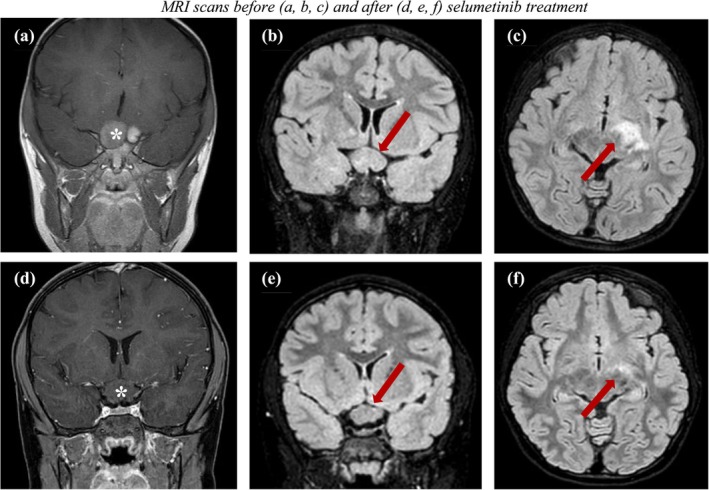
Pre‐selumetinib MRI: (a) coronal post‐contrast T1‐weighted, (b) post‐chemotherapy coronal and axial and (c) axial FLAIR images. Figure (a) demonstrates post‐contrast enhancement of the optic tract lesion; figure (c) shows signal hyperintensity of the optic tract. Two years after starting selumetinib: coronal contrast‐enhanced T1‐weighted (d), coronal FLAIR (e) and axial FLAIR (f) images demonstrate almost complete resolution of the left optic tract FLAIR hyperintensity, along with a slight reduction in the size of the optic nerve glioma and an absence of contrast enhancement.
